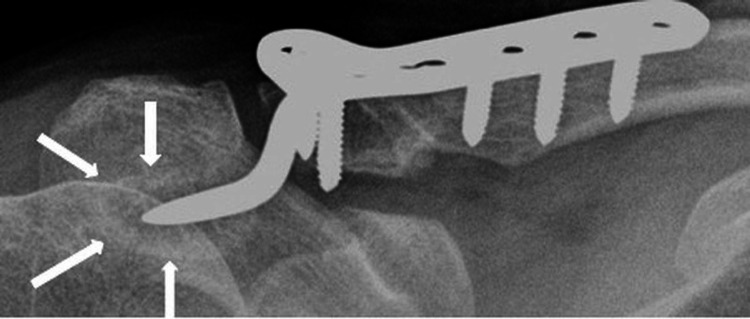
Radiograph showing subacromial osteolysis (arrows) after clavicular hook plate fixation.
